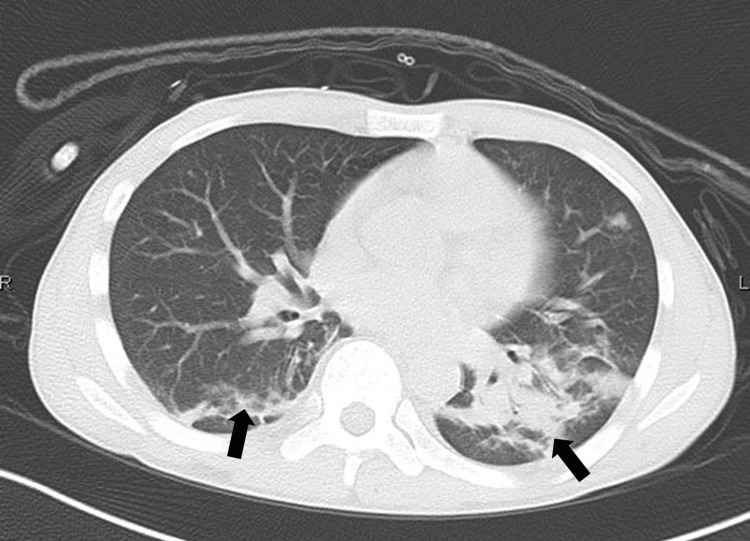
Chest CT (Case 2). Chest CT showing pneumonia with ground-glass opacities.
